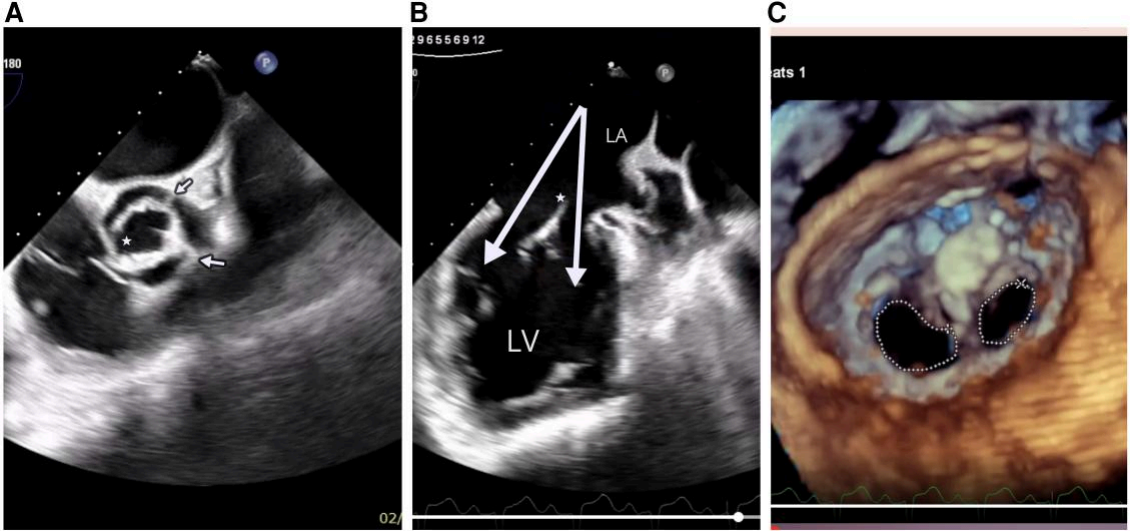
A*) Unicuspid unicommisural aortic valve with two raphes (arrows) and one commissure (star). *B*) Transoesophageal echocardiography showed double orifice mitral valve (arrows) with fibrous bridge (star). *C*) Asymmetrical double orifice mitral valve 3D reconstruction.
